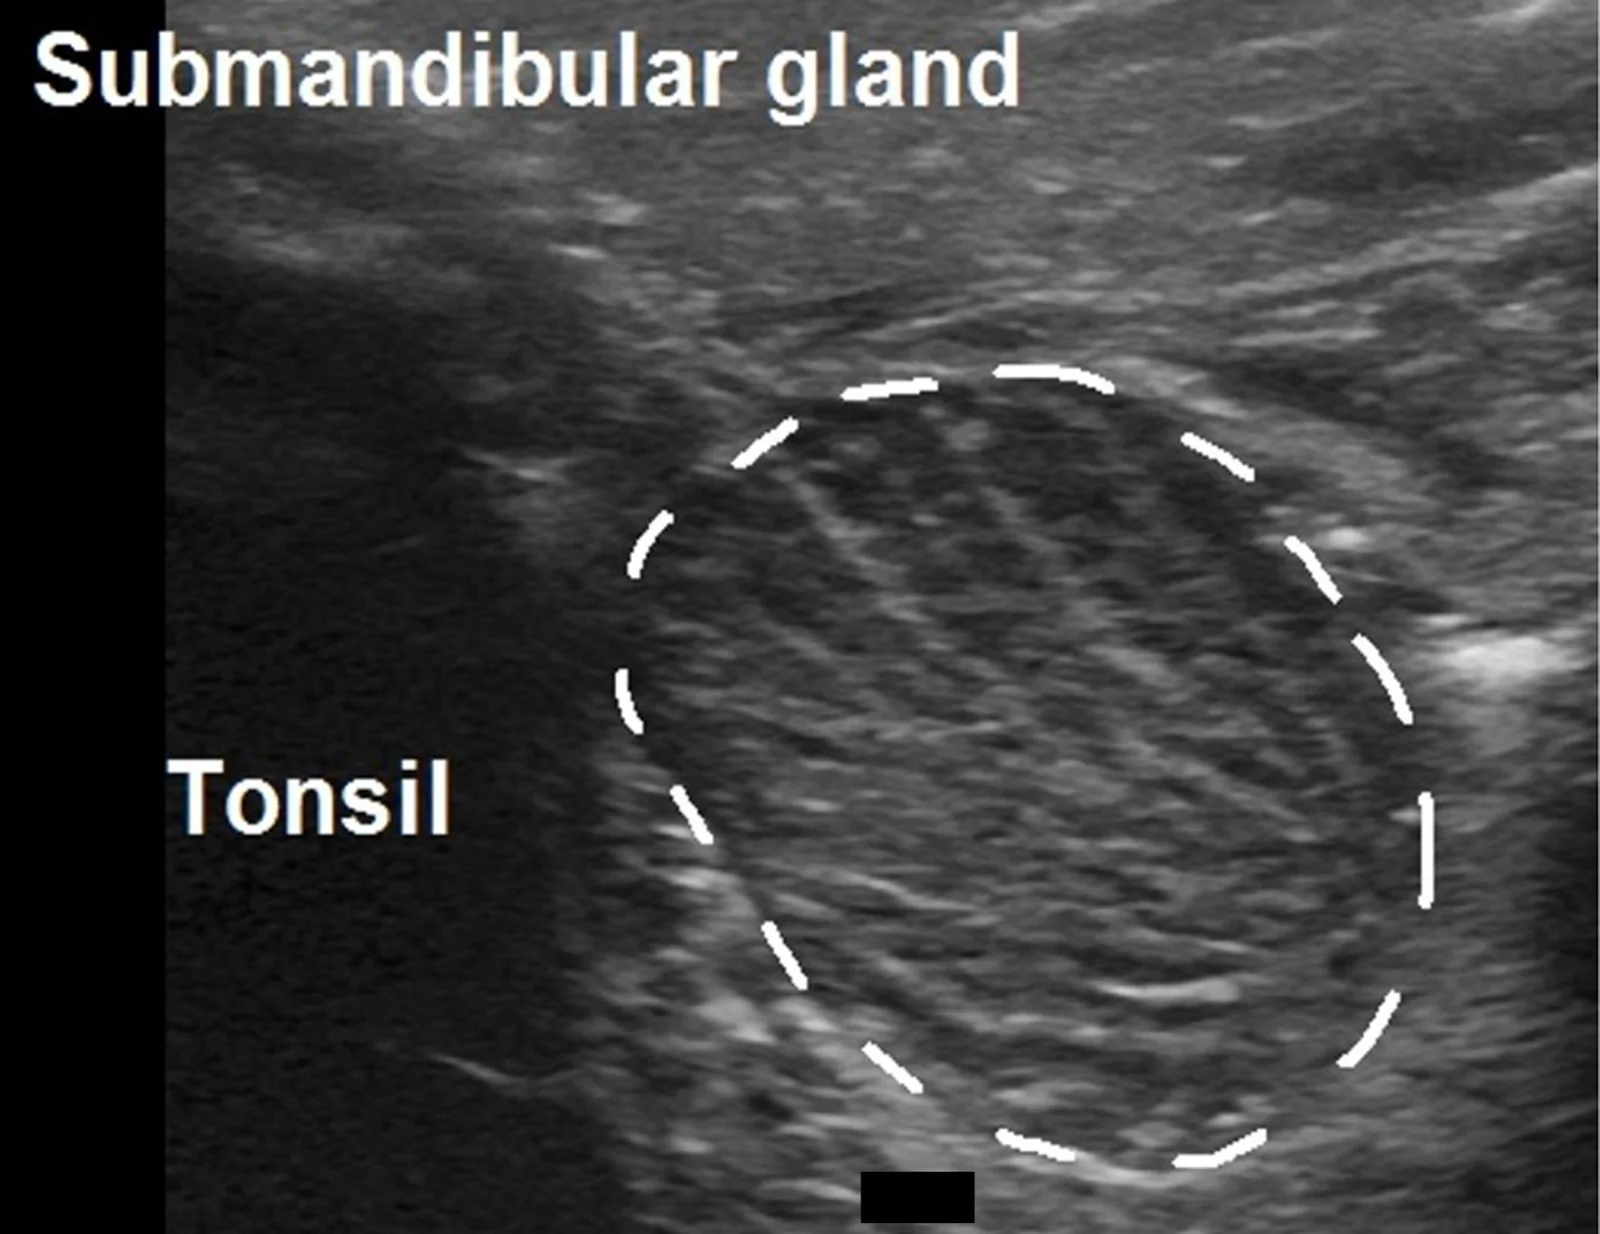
Submandibular ultrasound showing identification of tonsillar size.
